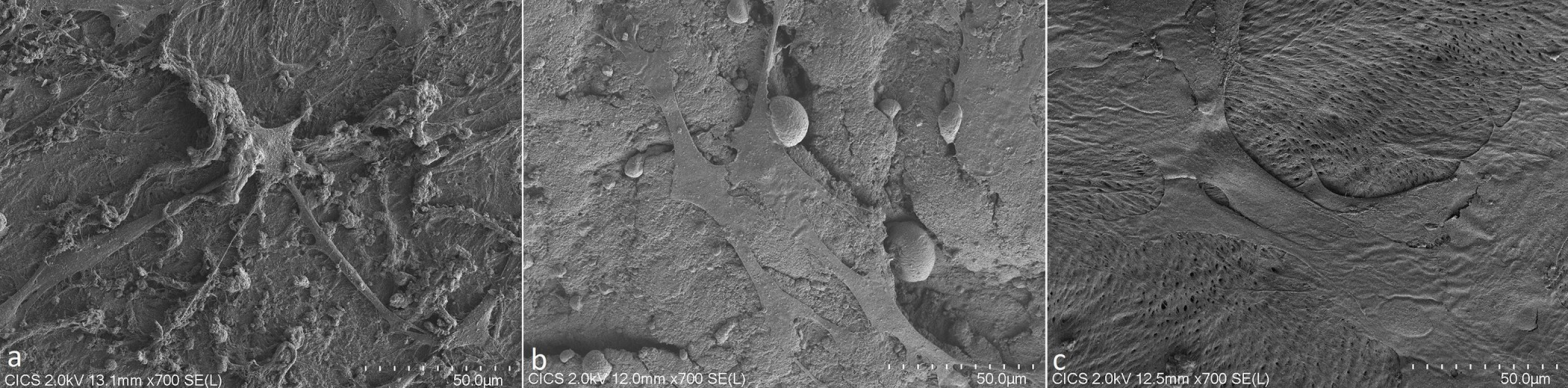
SEM images (x700 magnification) of osteoblasts on the 3 different bones after 4 days of culture (a: « F-bone », b: « scCO2-bone », c: « CT-bone »).
